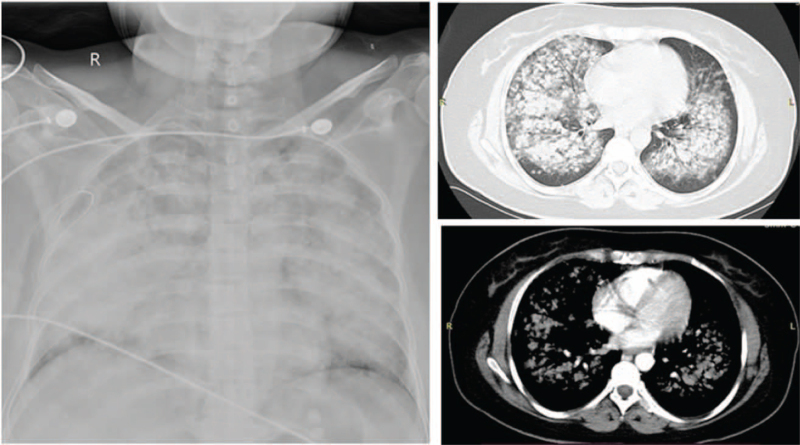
A chest radiography and computed tomography (CT) indicated multiple pulmonary nodules with air bronchograms, consolidation and solid pulmonary opacities.
